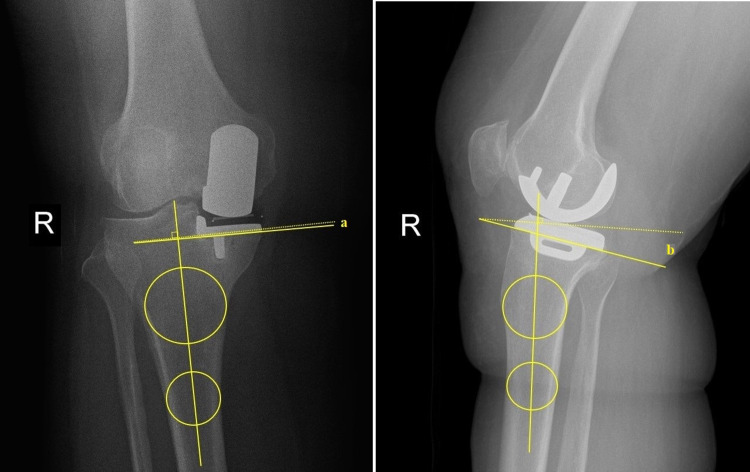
Standard anteroposterior and lateral views of an operated knee On the anteroposterior view, the tibial implant has to be perpendicular to the beam, thereby allowing a clear view of the joint space, and on the lateral radiographs, the femoral component has to be aligned to be perpendicular to the beam with the femoral condyles superimposed, and the tibial implant should be parallel to the horizontal plane. The coronal alignment of the tibial implants was measured with two circles drawn at a distance of 5 and 10 cm from the joint line and tangent to the medial and lateral cortex. A line passing between the midpoints of these circles and a line passing just underneath the tibial implant was used to measure coronal alignment (angle ‘a’). The sagittal alignment (slope) was measured with a line connecting midpoints of two intracortical circles at 5 and 10 cm distal to the knee joint and a line drawn just beneath the tibial implant (angle ‘b’).
